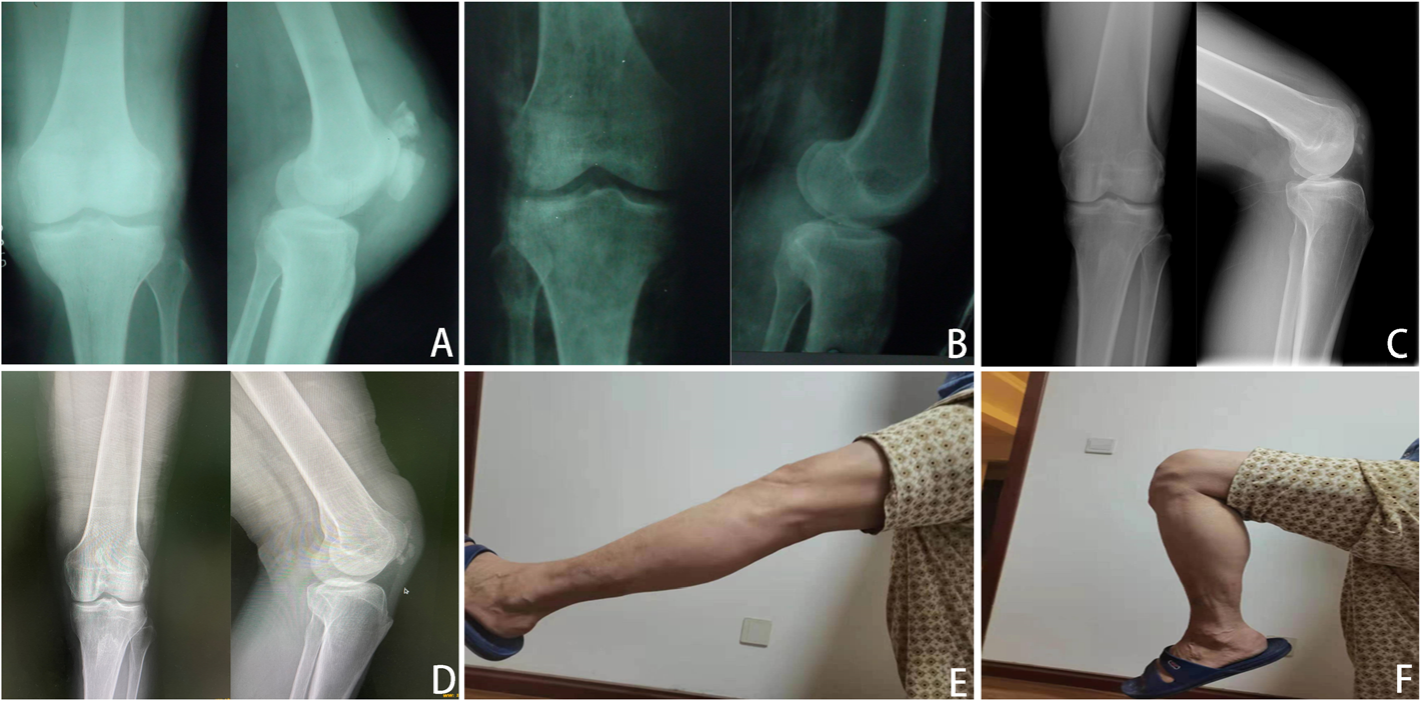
A Male, 57 years old, traffic accident, anteroposterior and lateral radiographs of the left knee showed patellar comminuted fractures preoperatively and B postoperative immediately, C postoperatively at 10 years with calcification developed. D Anteroposterior and lateral radiographs of the left knee postoperatively at the final follow-up. E Satisfactory functional outcome was restored normally at the final follow-up
